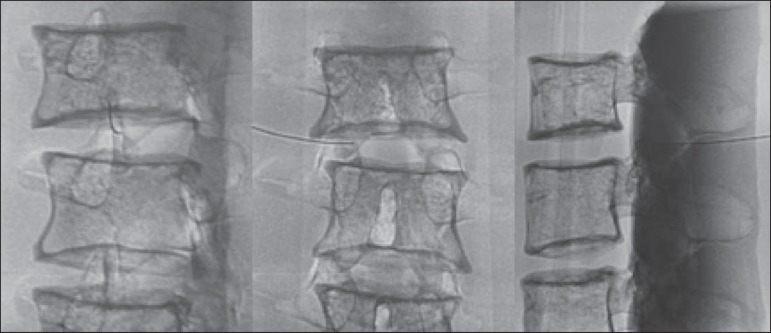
Radiographic appearance of an appropriate procedure.
